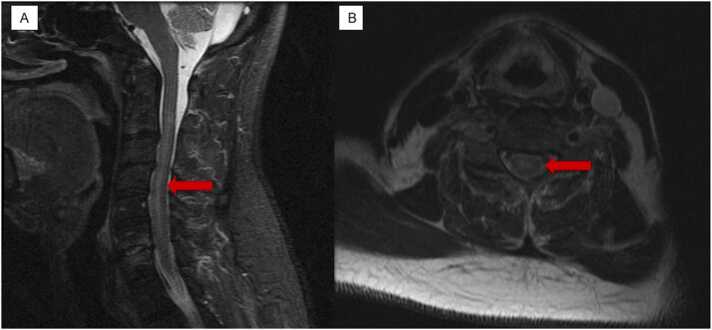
A. STIR sagittal cervical spine MRI with and without contrast. The red arrow shows edema within central cord. B. T2 Axial cervical spine MRI with and without contrast showing subtle hyperintensity without the spinal cord indicating spinal cord edema.
